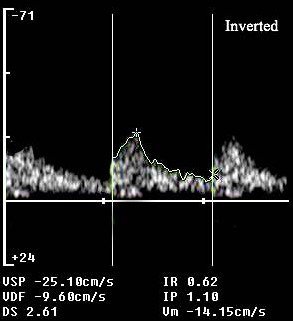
On patient presentation, the transcranial Doppler showed a dampened flow at the level of the basilar artery: a pulsatile flow with normal flow acceleration and decreased mean flow velocity (>30% difference between the proximal and distal arterial segments).
